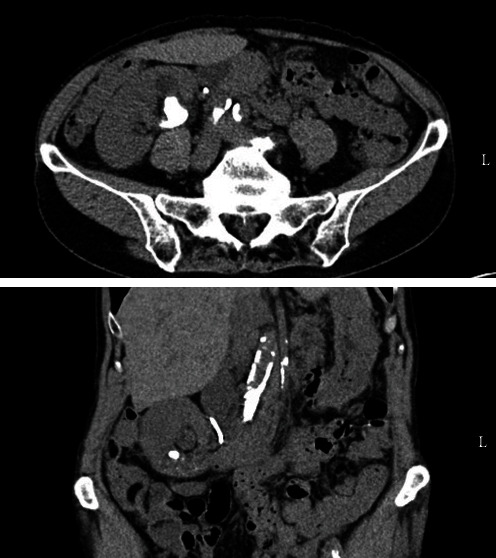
Representative images of CT scan of the abdomen and pelvis showing proximity of bowel to the right kidney. CT, computed tomography.
